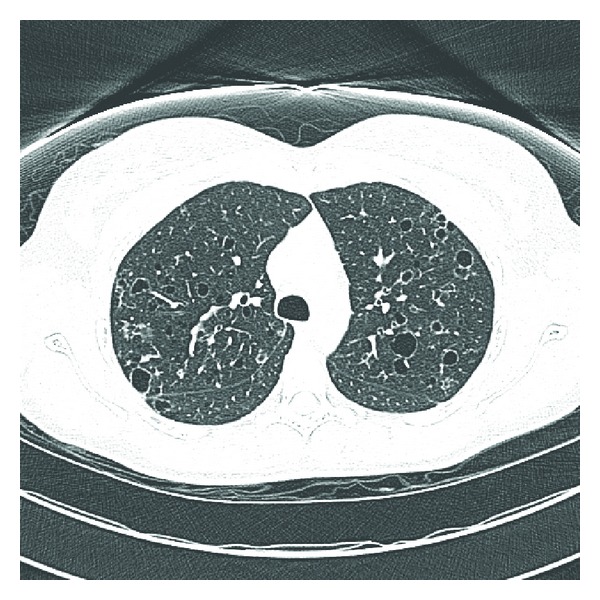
High-resolution chest CT.
